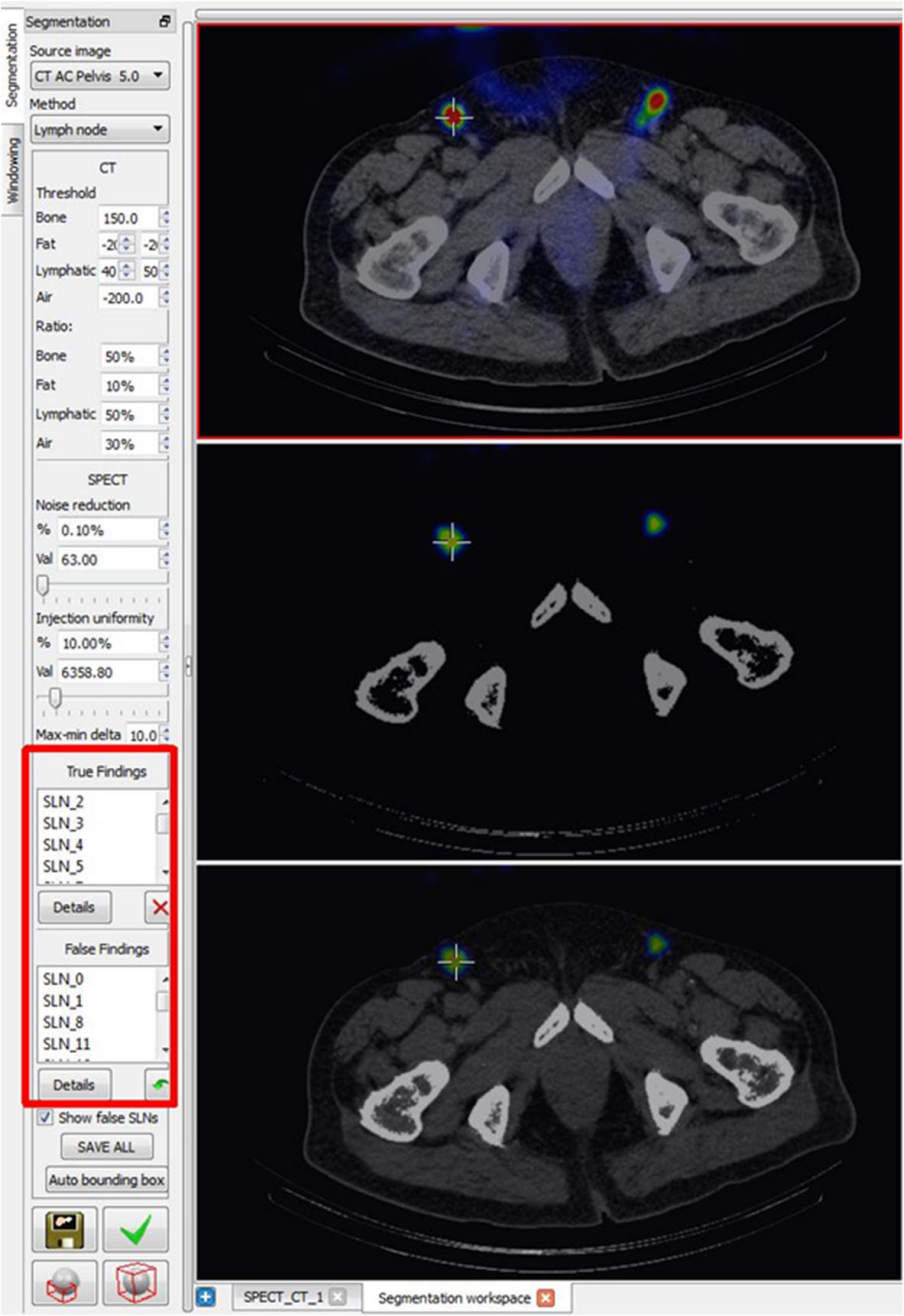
Excerpt from the screen display of the software (InterView FUSION/Mediso) with axial CT scan of the abdomen. The hot spot marked with a cross on the *right* inguinal region was identified as a “true” finding by the software and thus as a true SLN. On the *lower left* periphery of the image you can find the lists of the “true” and “false” findings (*red frame*)
